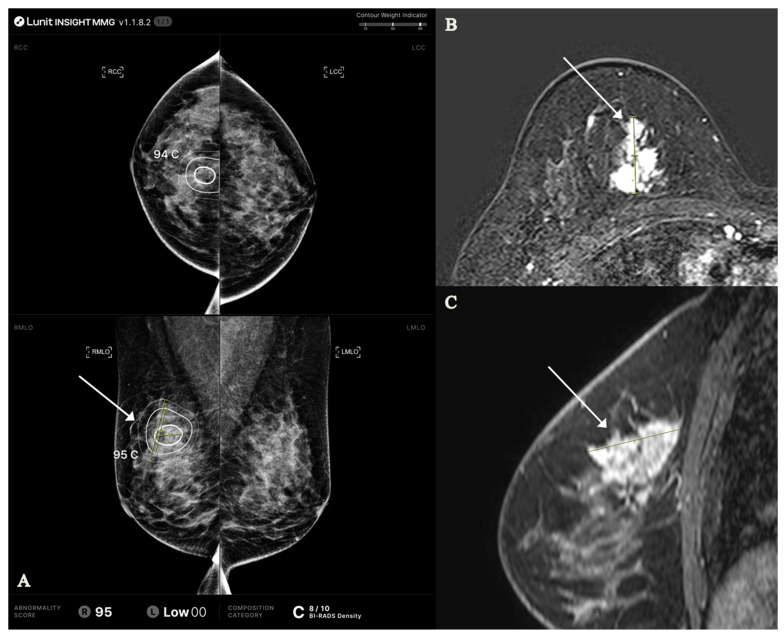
AI-CAD mammography and MRI findings in a 48-year-old woman with invasive ductal carcinoma, grade II (HR+/HER2+, Ki-67 index 34%). (A) AI-CAD mammography shows heterogeneously dense breasts (category C, density score 8/10) and a high abnormality score (95) on the right mediolateral oblique view. The lesion was classified as calcification. Tumor size along the inner, middle, and outer contours was 2.0, 3.5, and 4.9 cm, respectively (T1, T2, T2). (B,C) Contrast-enhanced MRI reveals a heterogeneously enhancing mass with non-mass enhancement in the corresponding area. The lesion measured 3.3 cm on axial and 3.7 cm on sagittal views; the maximal diameter (3.7 cm) was used for T2 staging. Arrows indicate the lesion; yellow lines represent caliper-based measurements. AI-CAD = artificial intelligence-based computer-aided detection; HR = hormone receptor; HER2 = human epidermal growth factor receptor 2.
